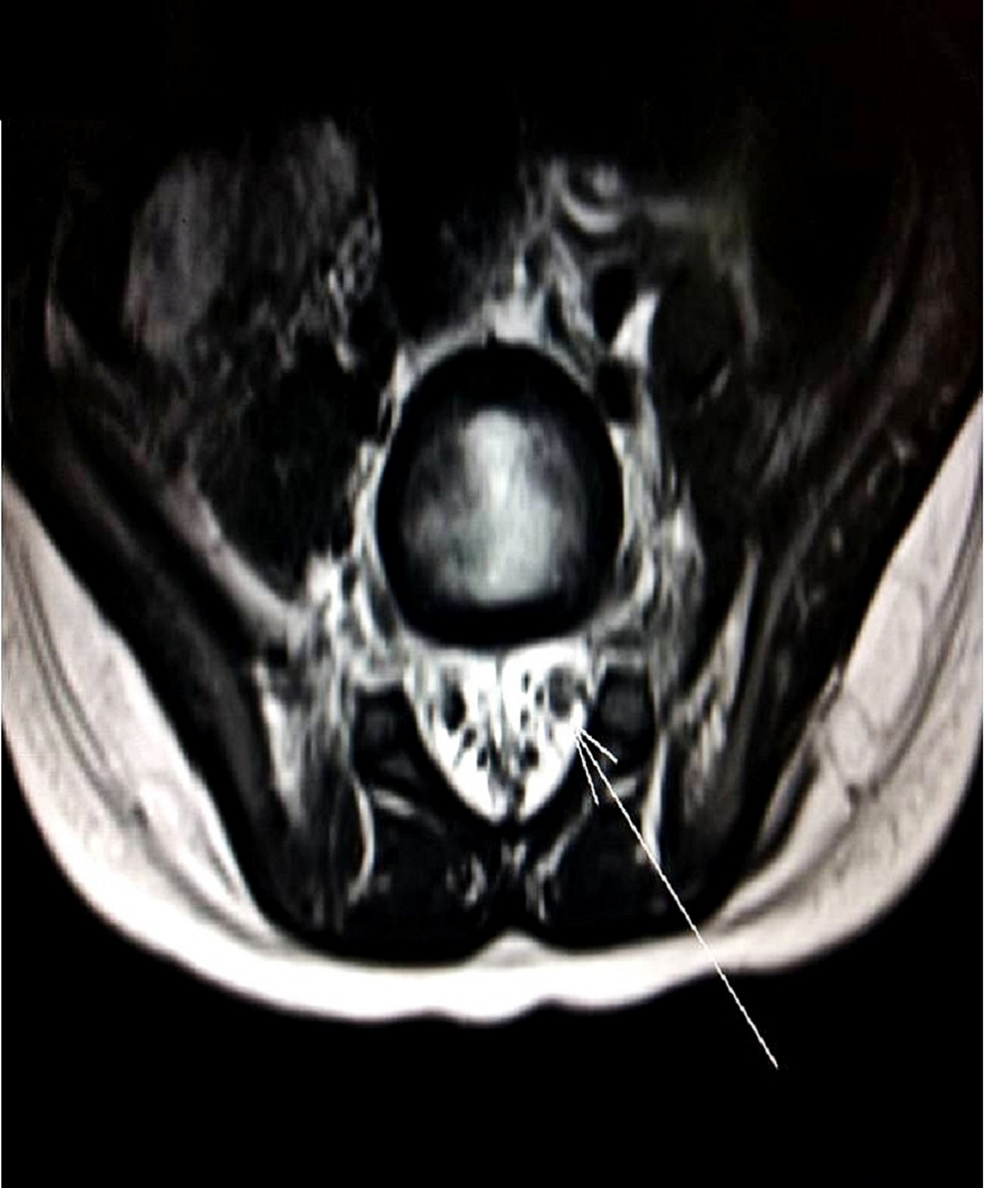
Axial section MRI spine showing thickened nerve root with intense post-contrast enhancement MRI: magnetic resonance imaging
